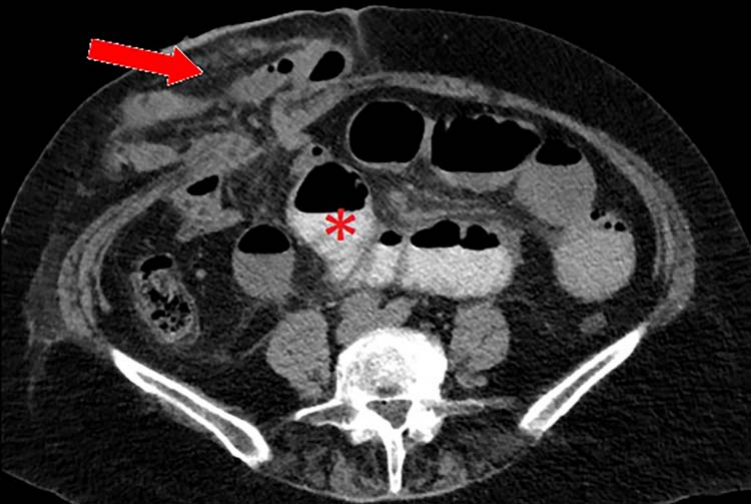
Axial CT scan with oral contrast demonstrating right-sided parastomal hernia containing small bowel loops (arrow) and proximal small bowel dilatation (asterisk).
